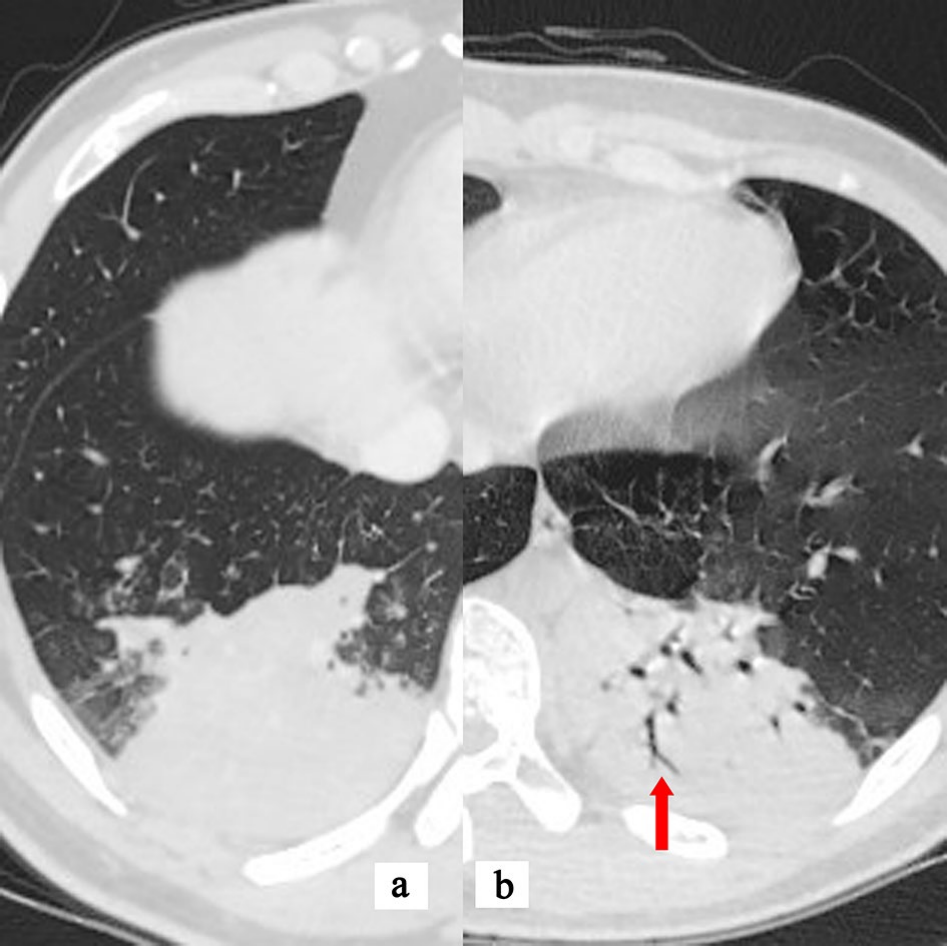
(a) HRCT findings of a 38-year-old male case with mycoplasma pneumoniae pneumonia. (b) HRCT findings of a 33-year-old male case with pneumococcal pneumonia. The red arrow showed the air-bronchogram.
